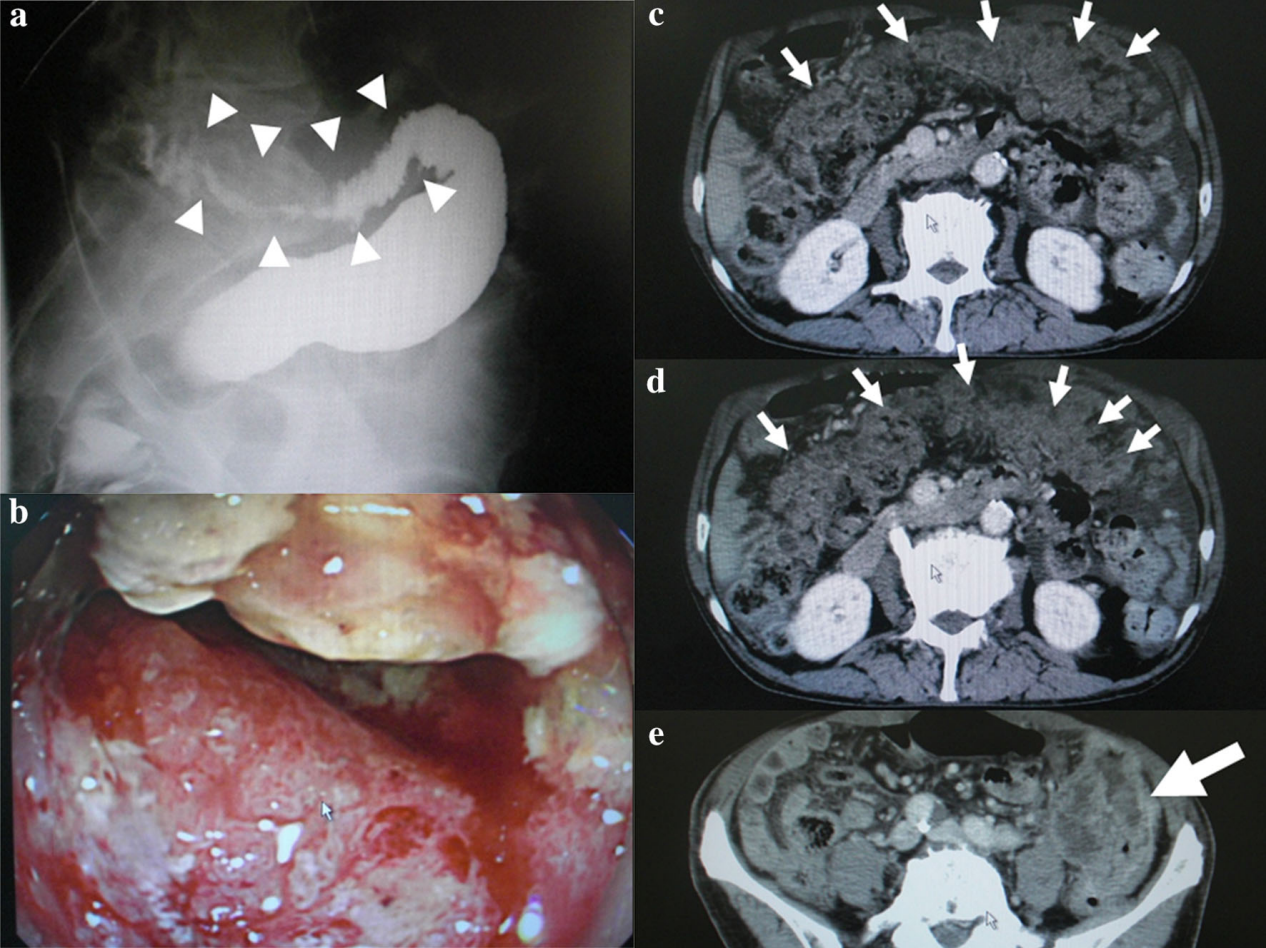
Findings of barium enema, colonoscopy, and abdominal computed tomography. a A severely stenotic lesion (*white arrowheads*) was observed in the descending colon just proximal to the sigmoid-descending junction. b A large circular type 2 lesion was detected by colonoscopy and caused severe bowel stenosis. c, d Greater omentum was occupied with numerous peritoneal nodules (*black arrows*) and formed an omental cake. Moderate ascites was identifiable. e Primary lesion was easily detectable as a large mass by computed tomography (*large white arrow*)
